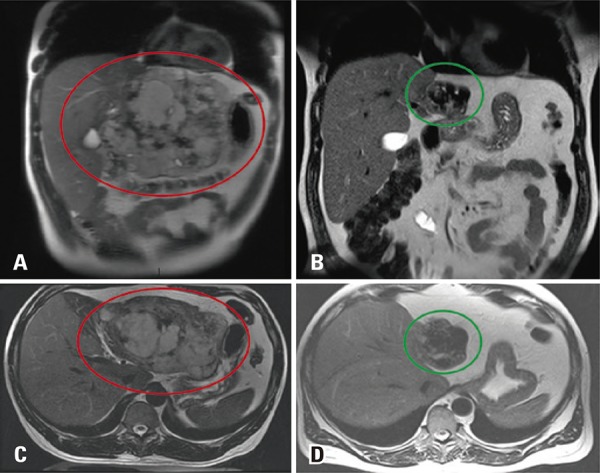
Giant hyalinized hemangioma. Magnetic coronal and axial resonance in T2-weighted image showing characteristic high signal (A and C), and posterior signal and volume reduction after 6 years (B and D) – 17cm to 8cm
